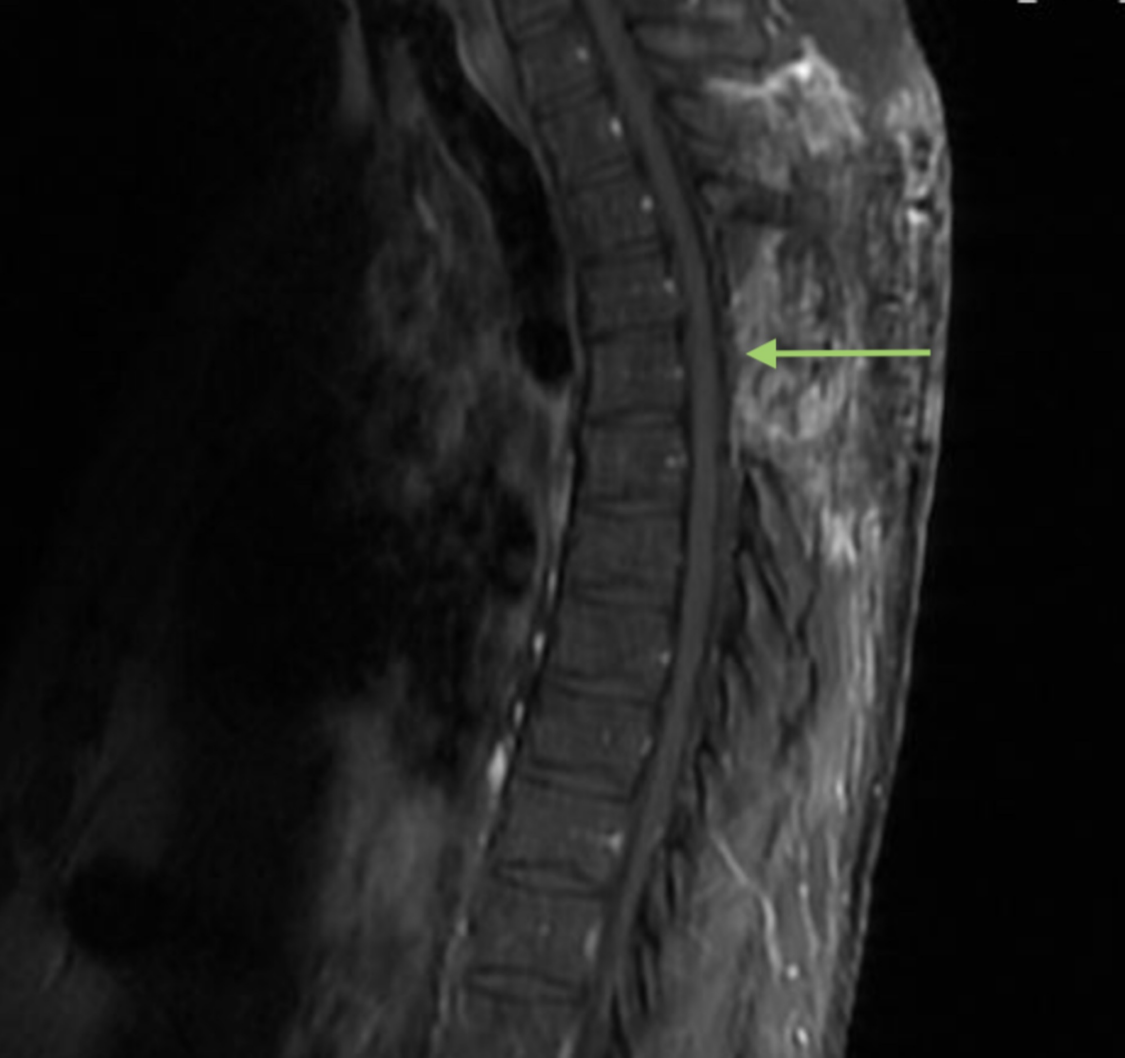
Post-operative MRI thoracic spine sagittal T1 fat suppression with contrast confirms gross total resection as indicated by the arrow.
